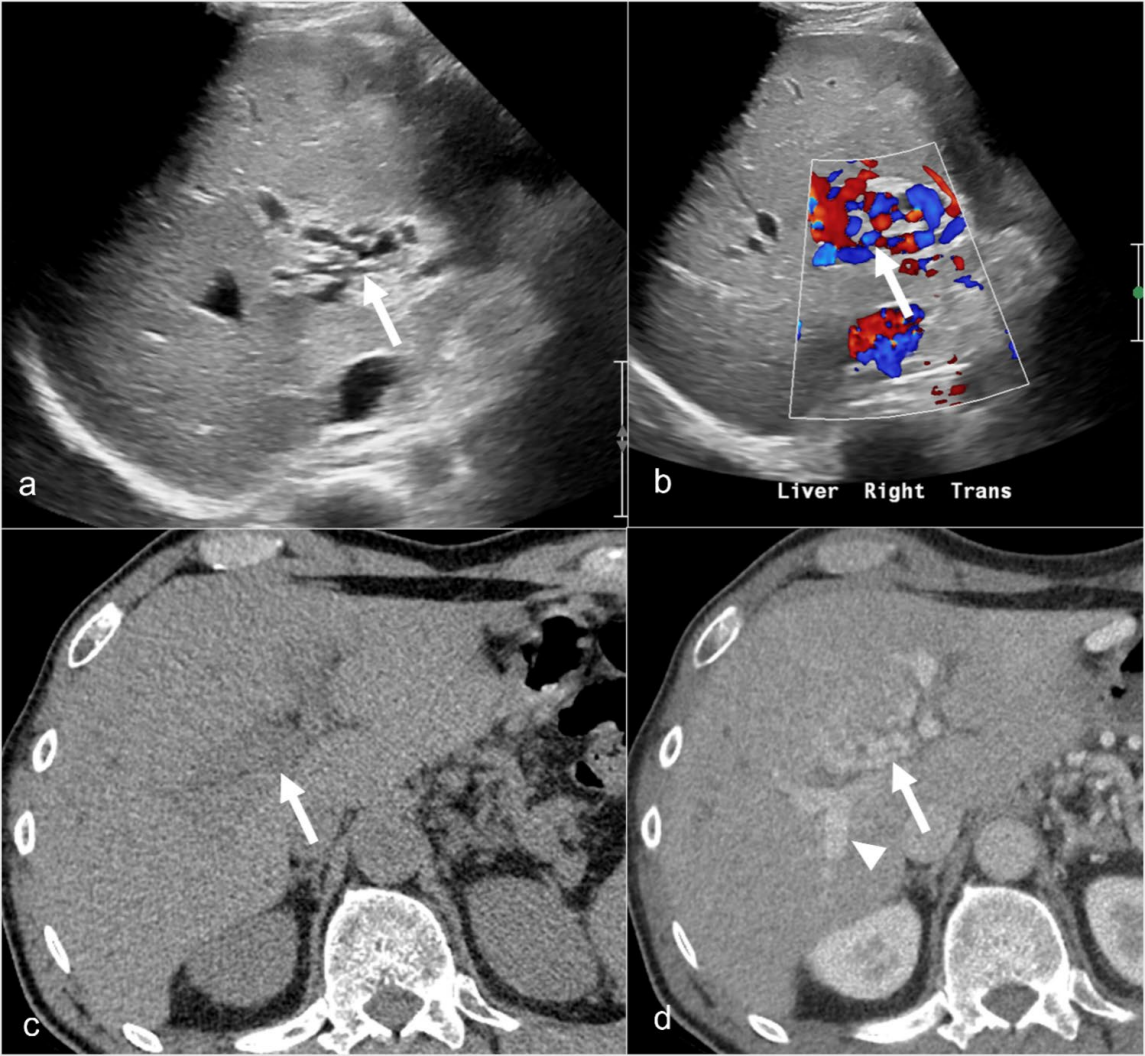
Cavernous transformation of the portal vein in a 34-year-old male patient with recurrent abdominal pain. Grayscale (a) and color Doppler (b) Ultrasound image demonstrate anechoic tortuous appearing cystic lesion (arrow). Blood flow is present on color Doppler (arrow). Axial non-contrast CT (c) demonstrates low density area in the region of portal vein (arrow). Axial contrast-enhanced CT (d) demonstrates tortuous veins secondary to cavernous transformation of the portal vein in the setting of necrotizing pancreatitis.
